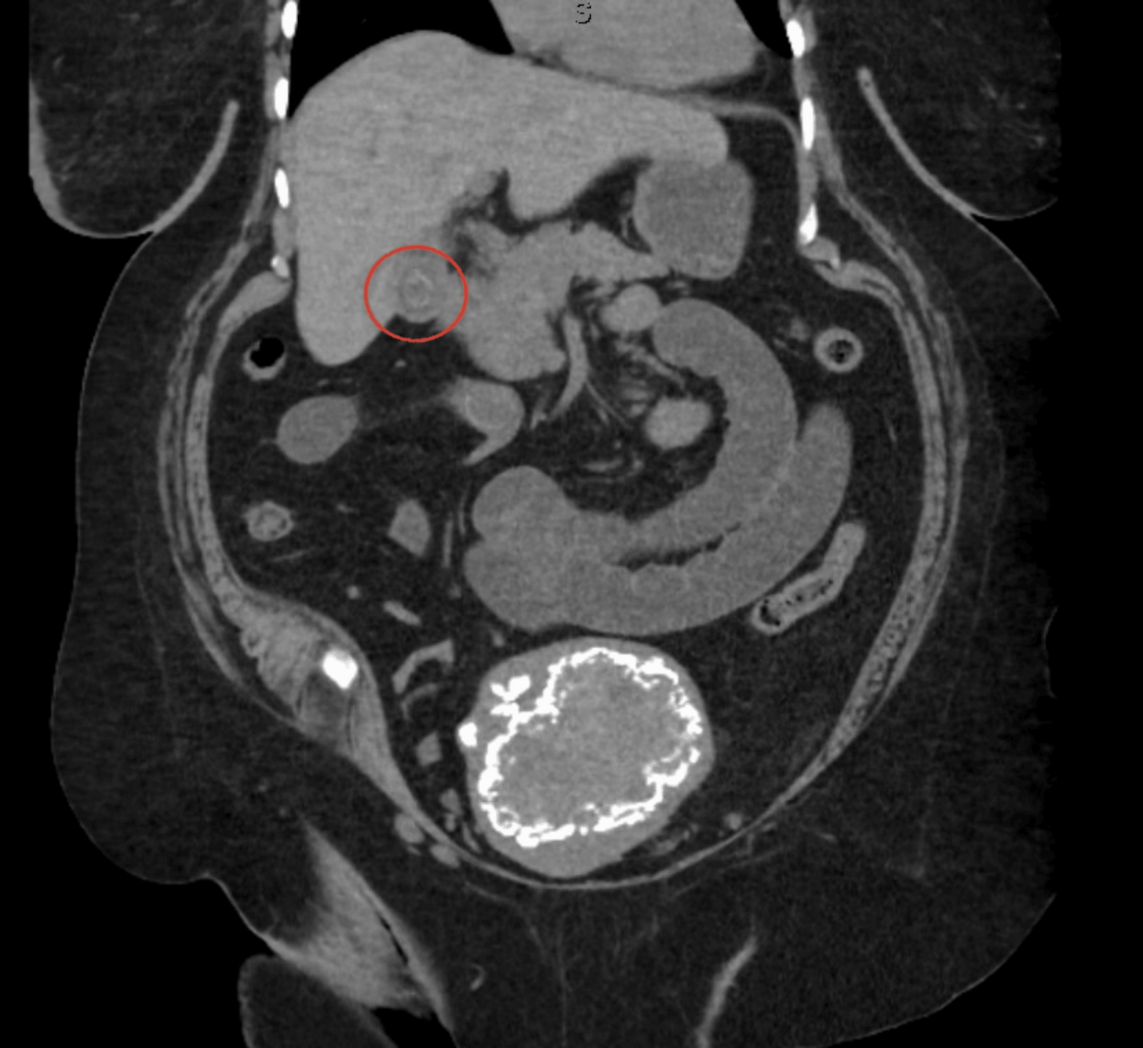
CT demonstrating cholecystoenteric fistula with a remaining stone in the gallbladder (circled in red)
